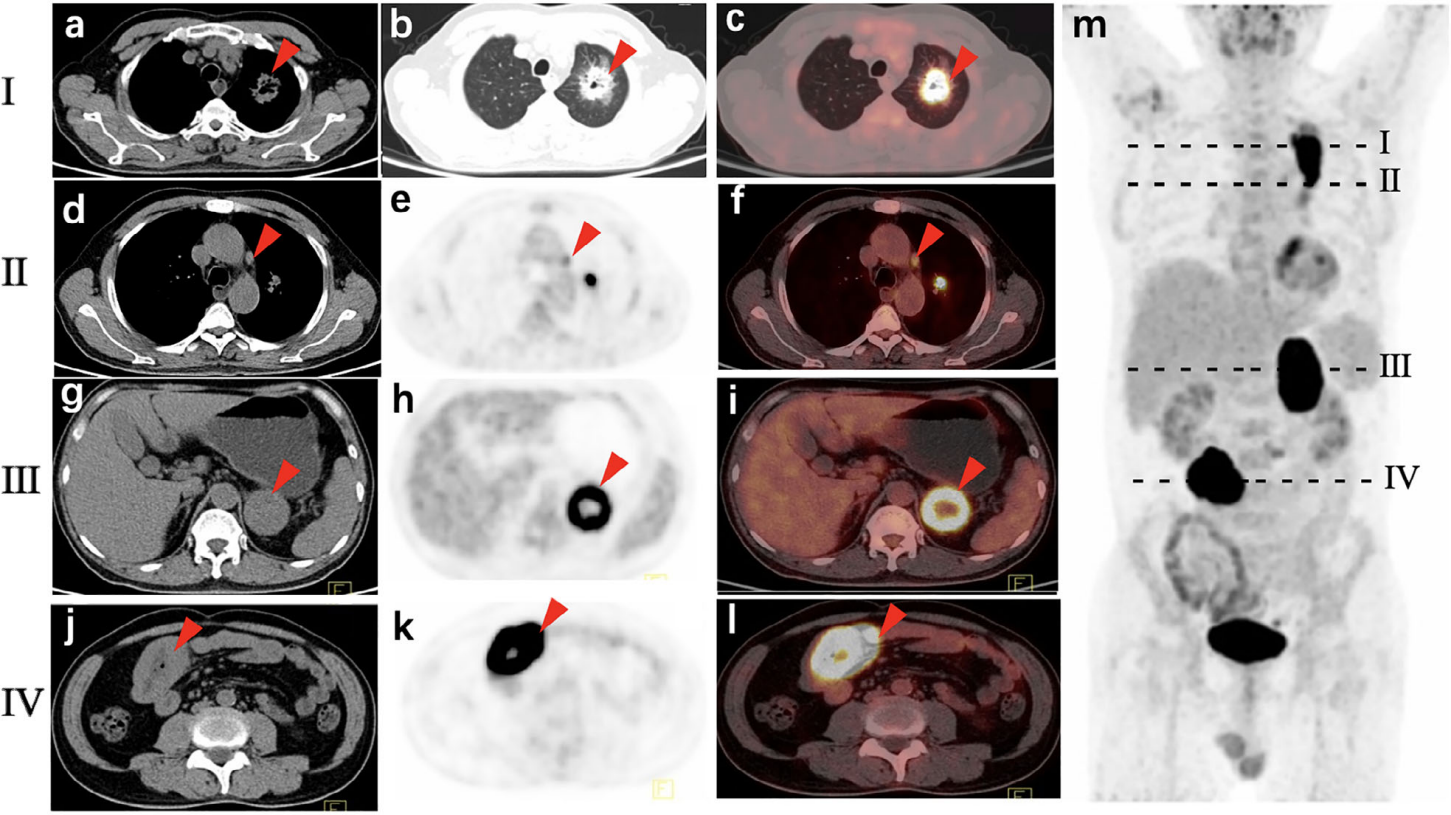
^18^F‐FDG PET/CT images of Case 1. I. (a) Soft tissue window; (b) lung window; and (c) PET/CT fusion image of left lung lesion (arrow) showed an irregular mass with lobulation, burr, central cavity and annular marked FDG uptake with maximum standardized uptake value (SUVmax) of 33.2. II. (d) Transaxial CT; (e) PET; and (f) PET/CT fusion image of a mediastinal lymph node measuring 1.5 × 0.9 cm with a SUVmax of 3.4. III. (g) Transaxial CT; (h) PET; and (i) PET/CT fusion image of left adrenal gland lesion showed a round mass measuring 7.6 × 5.1 × 5.0 cm, characterized by obvious necrosis with remarkably high FDG uptake (SUVmax:30.8). IV. (j) Transaxial CT; (k) PET; and (l) PET/CT fusion image of the intestinal lesion showed thickening intestinal wall up to 2.5 cm thickness and 7.3 cm long, with SUVmax of 41.6 in the right middle abdomen. No intestinal stenosis was observed. (m) Maximum intensity projection image of whole body showed high uptake lesions (red arrow) in (I) left thorax; (II) mediastinal lymph node; (III) left adrenal area; and (IV) right middle abdomen.
